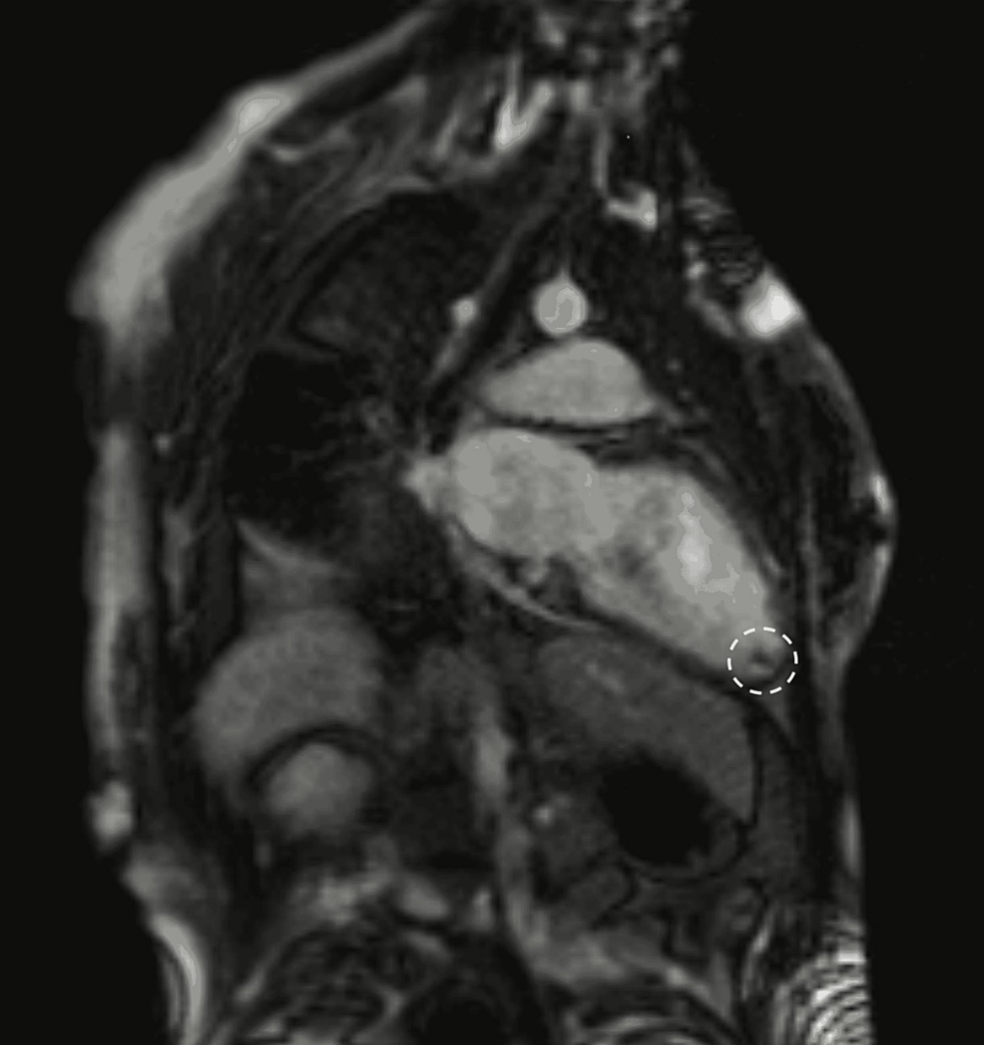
Cardiac MRI showing apical left ventricular thrombus (white circle).
